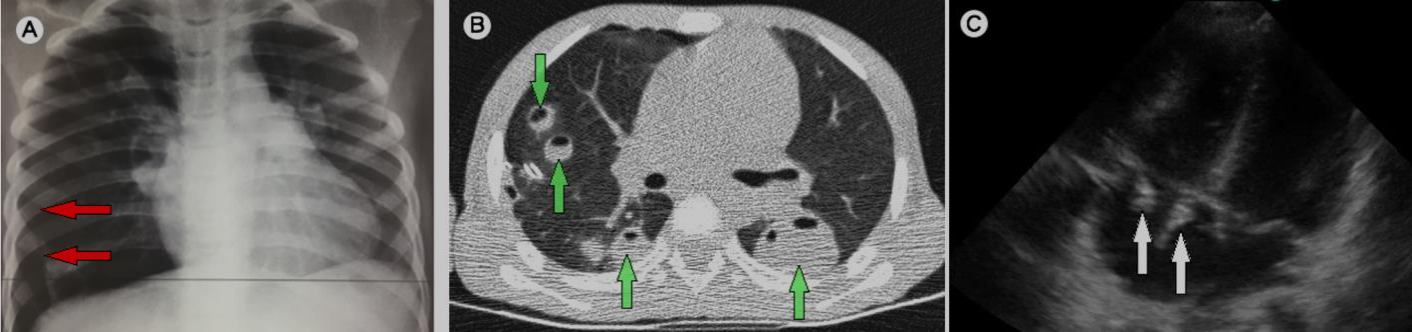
Imaging findings in a right-sided endocarditis with spontaneous pneumothorax and septic pulmonary emboli: (A) chest radiograph at admission, showing a right-sided hemithoracic pneumothorax (red arrows) and a partially-collapsed right lung; (B) lung CT scan on day 1: multiple, differently-sized nodules with cavities in the left and right lungs (green arrows) indicative of septic pulmonary emboli; (C) an apical four-chamber-view transthoracic echocardiograph showing tricuspid valve vegetations in the right atrium during systole (white arrows)
